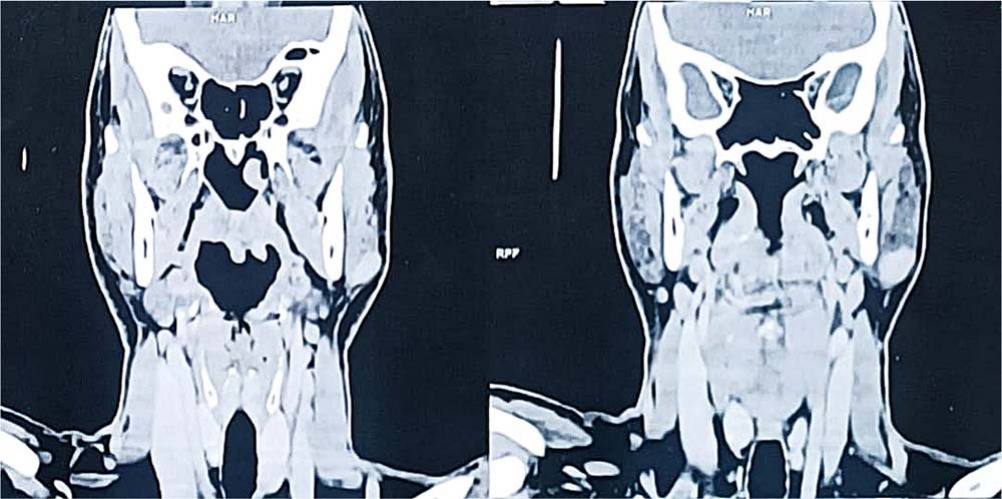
Coronal contrast-enhanced CT scan confirming the presence of an oval, homogeneous, enhancing lesion in the left parotid gland.
